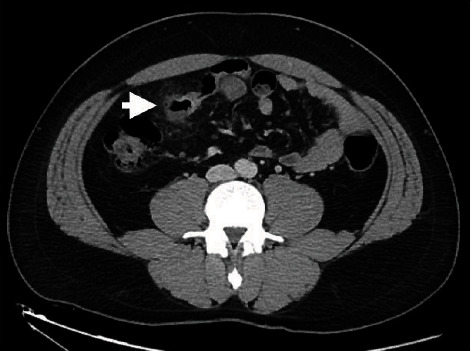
Abdominal computed tomography showing a blind-ending gas-filled structure with surrounding fat stranding and in continuity with small bowel from the antimesenteric border of the ileum (arrow).
